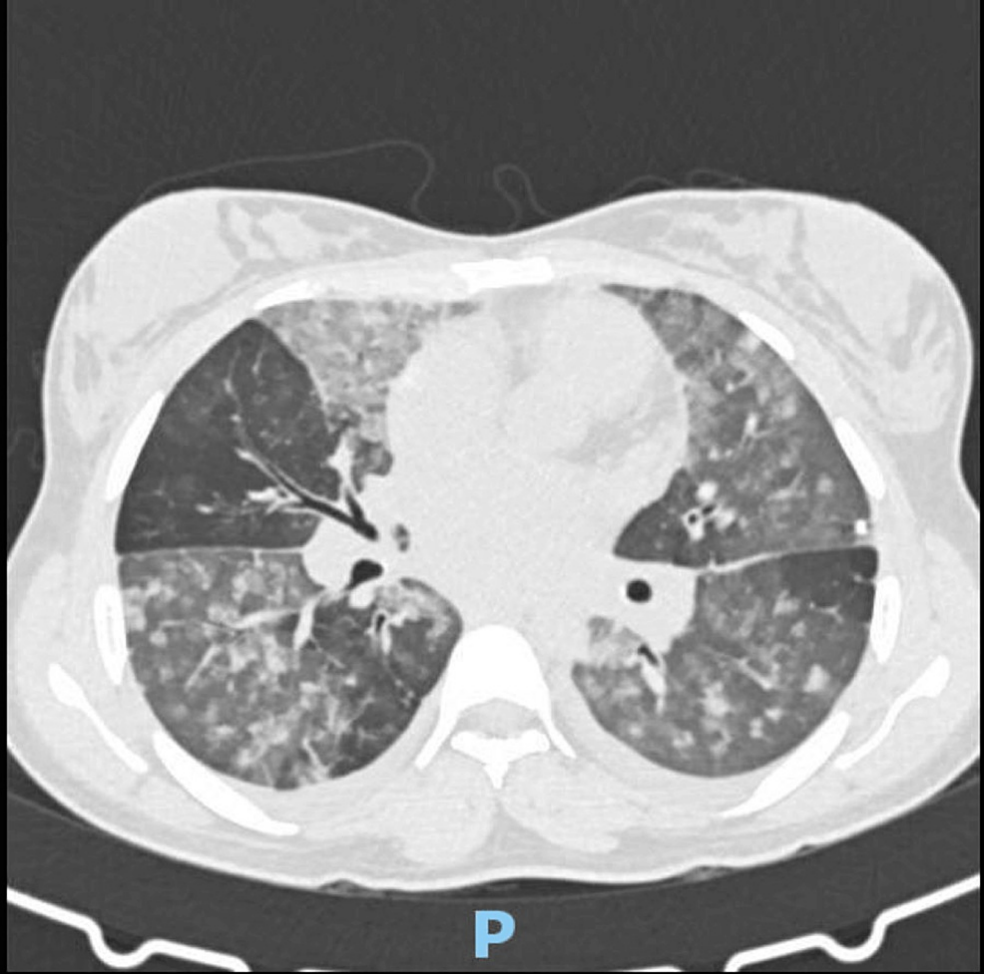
Thoracic CT scan on admission Thoracic CT scan showing extensive infiltrate in both lungs with areas of ground glass opacification and mild pleural effusions.
